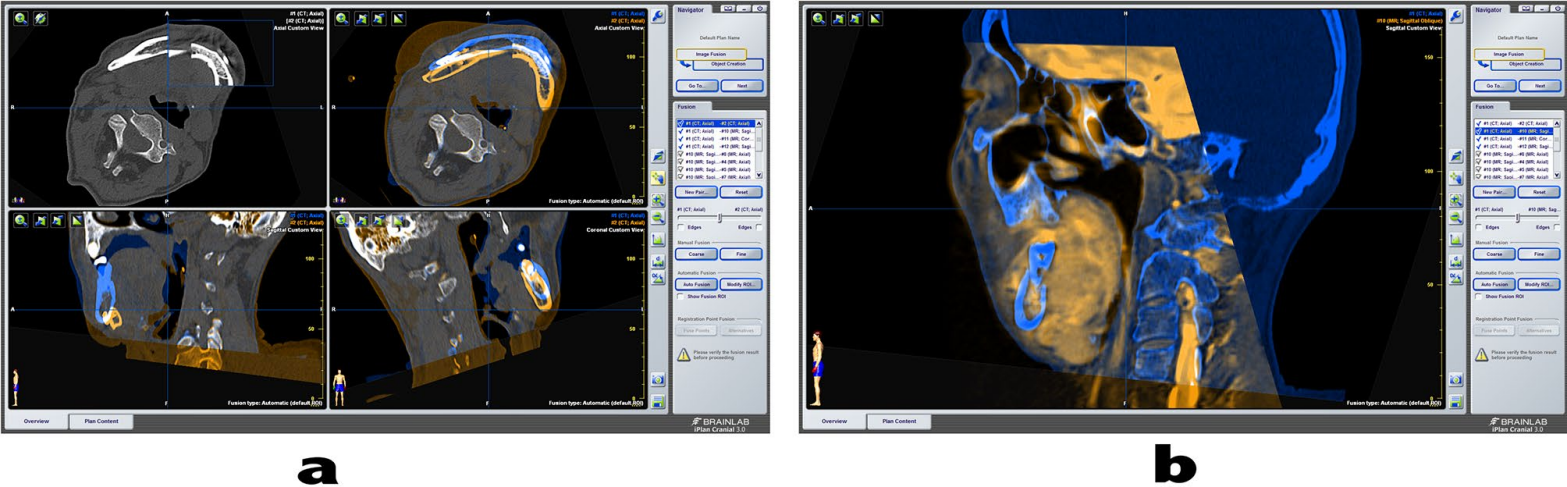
Image fusion is performed by computational software. a The preoperative and postoperative CT images were fused to show that the position of the mandible was different. b CT and MR image matching fusion
